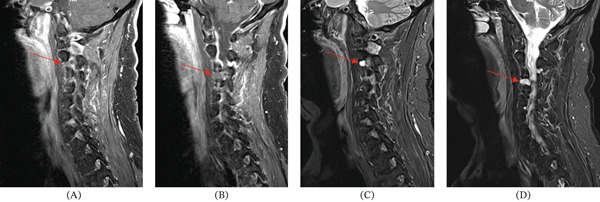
These MRI sequences of the cervical spine in our patient show T2‐hyperintense, nonenhancing lesions at the right C2–C3 uncovertebral joint region and left C3–C4 uncovertebral joint region. (A,B) Panels A and B are T1‐weighted fat‐saturated images showing the lesions (red arrows) at C2–C3 and C3–C4, respectively. (C,D) Panels C and D are T2‐weighted STIR images showing the lesions (red arrows) at C2–C3 and C3–C4, respectively.
